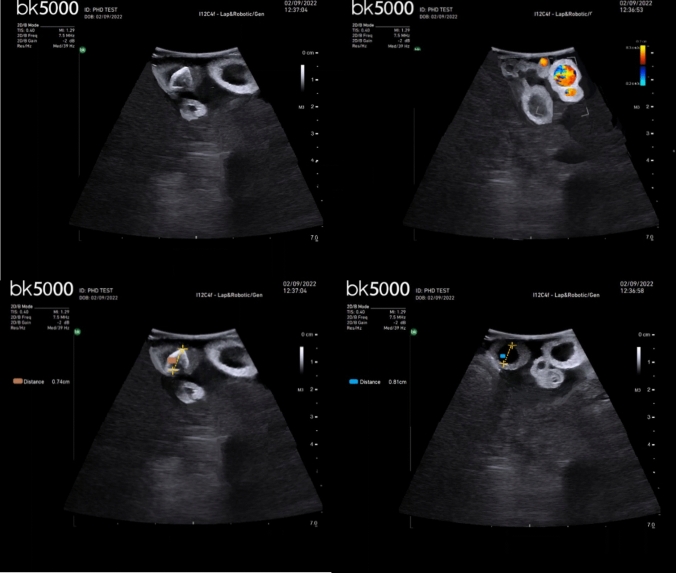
Creation of a dataset of ultrasound images in B-mode (upper left), Doppler mode (upper right), and with measures of the anatomical features (lower left and right)
